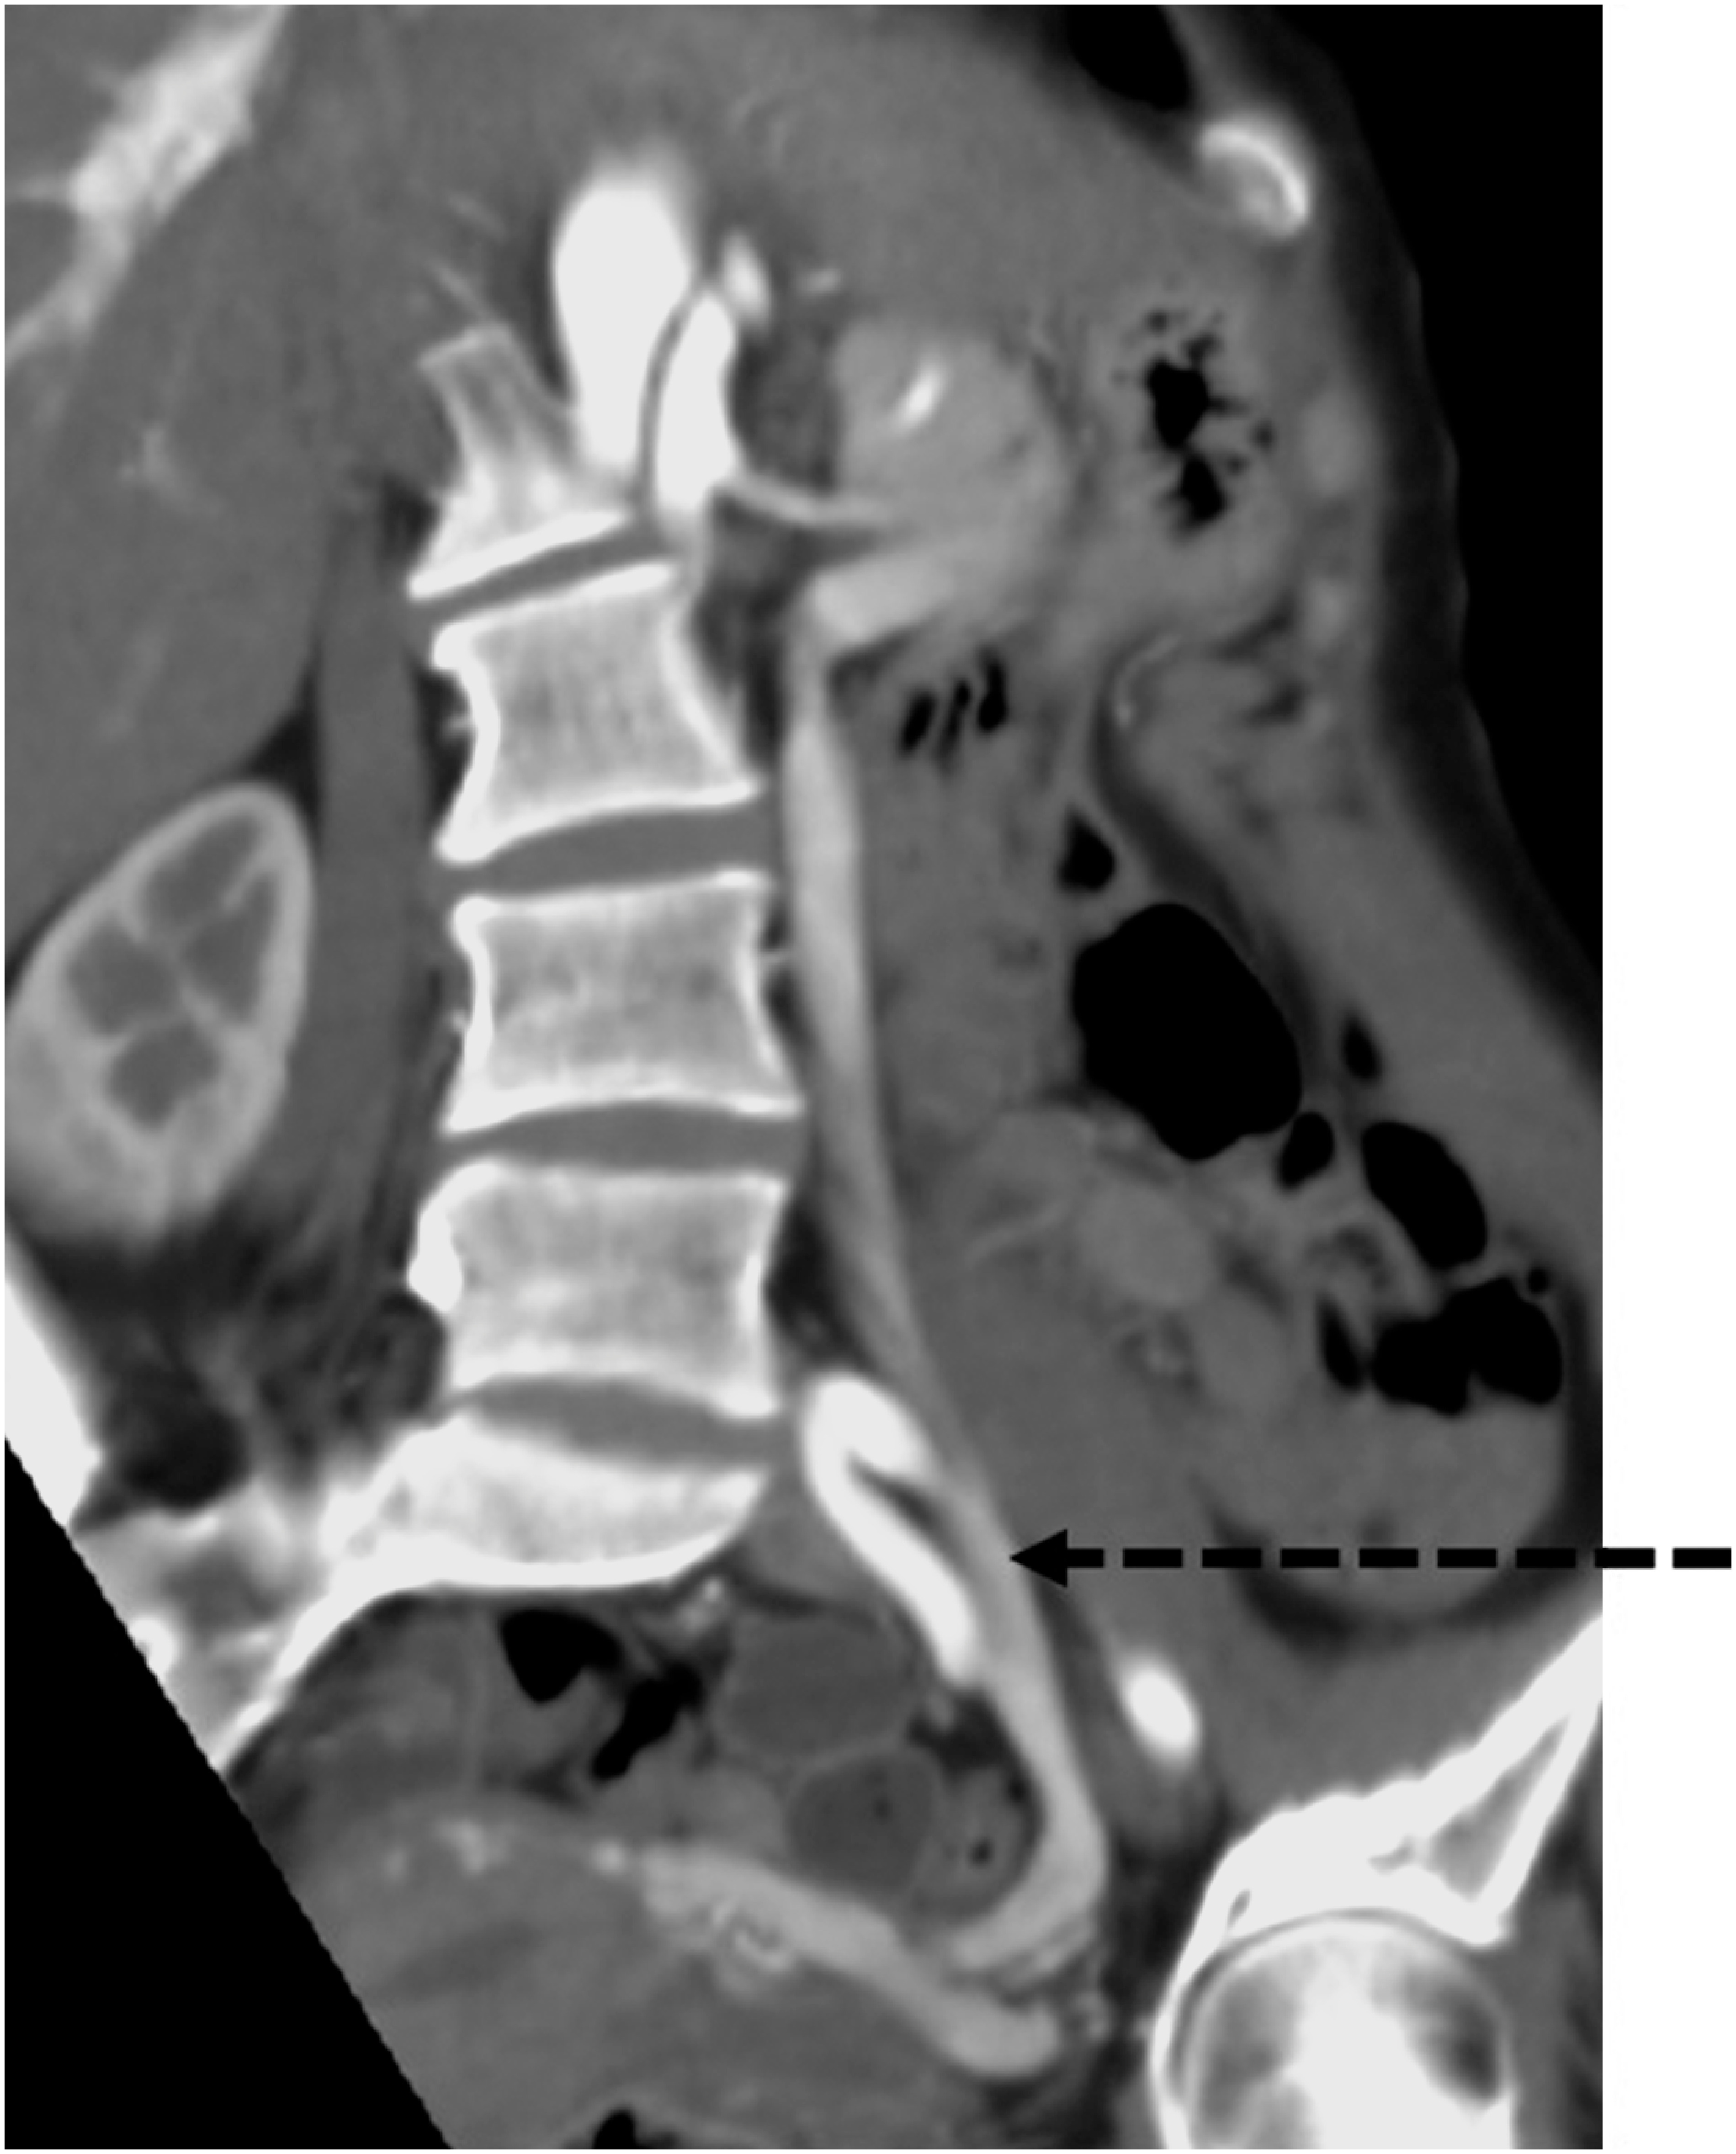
Fig. 3 A postoperative computed tomographic scan revealing mild dilation of the left gonadal vein (dotted arrow).
